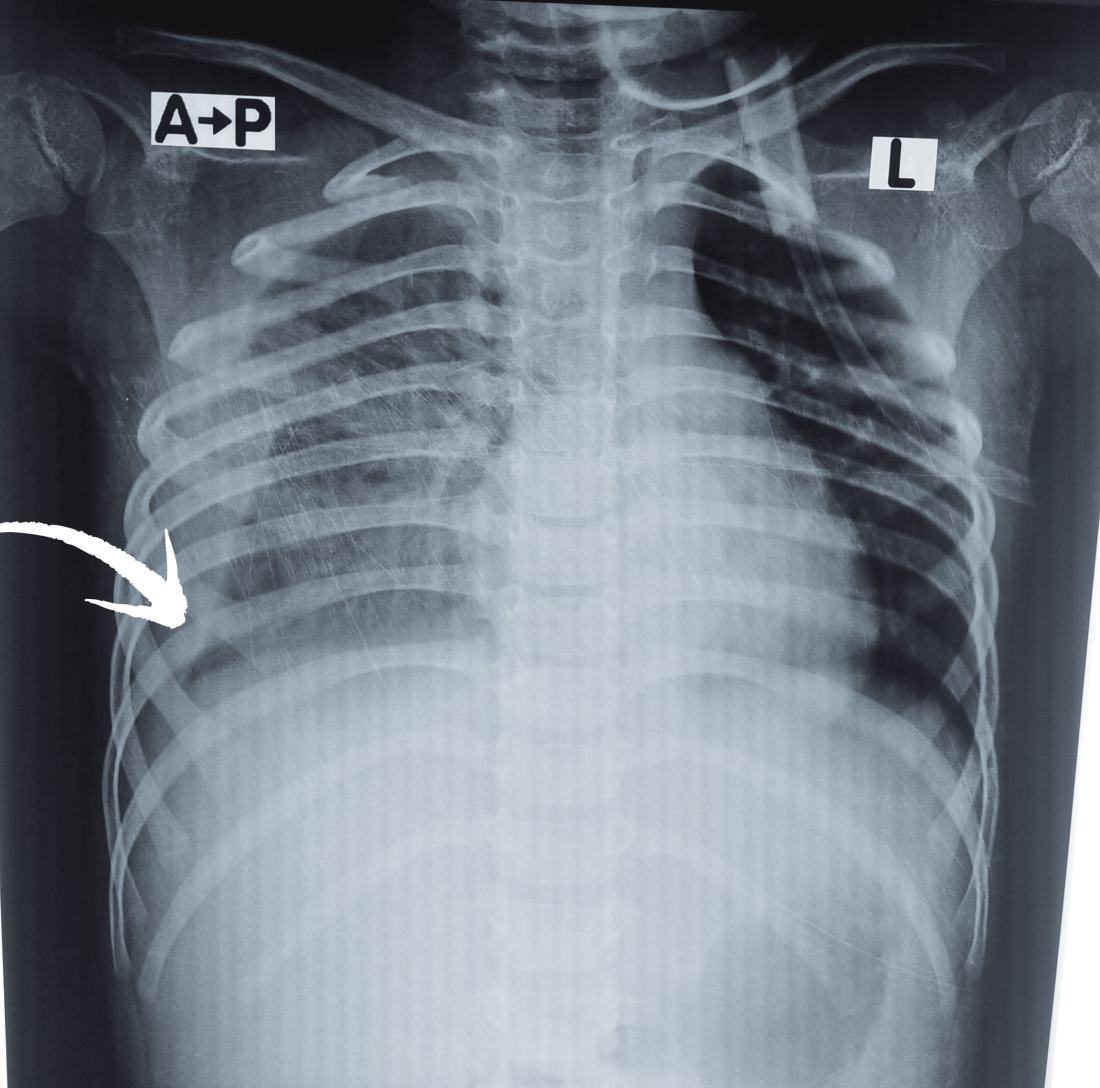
Chest radiograph demonstrating moderate right-sided pleural pleural effusion (~250 mL). Note the blunting of the right costophrenic angle and homogeneous opacity in the lower lung zone.
